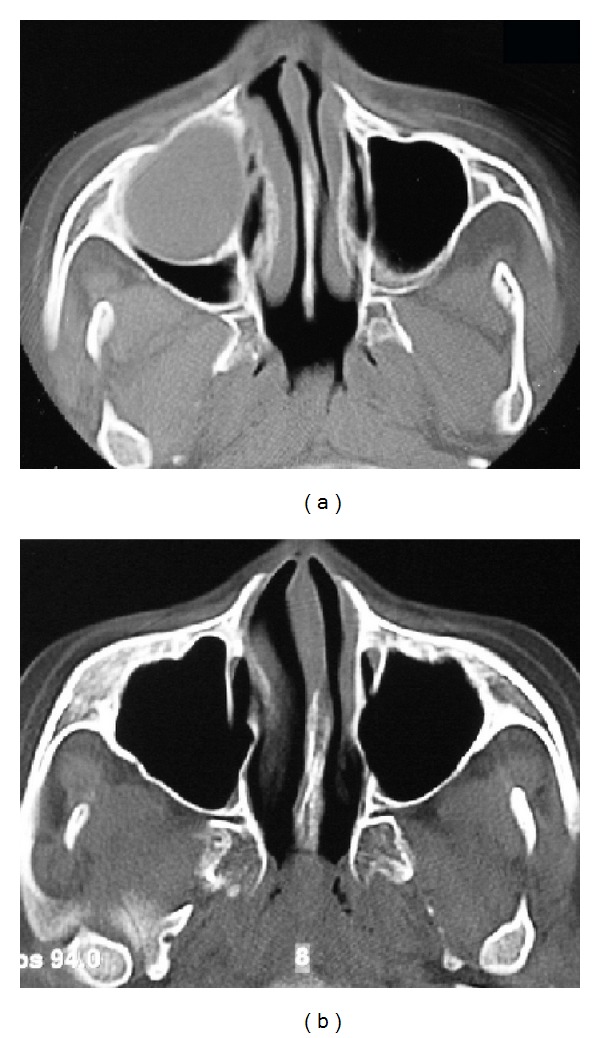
CT axial images showing opacity of the right maxillary sinus in the diagnostic phase (a); maxillary sinus cleared after 3 months of treatment (b).
